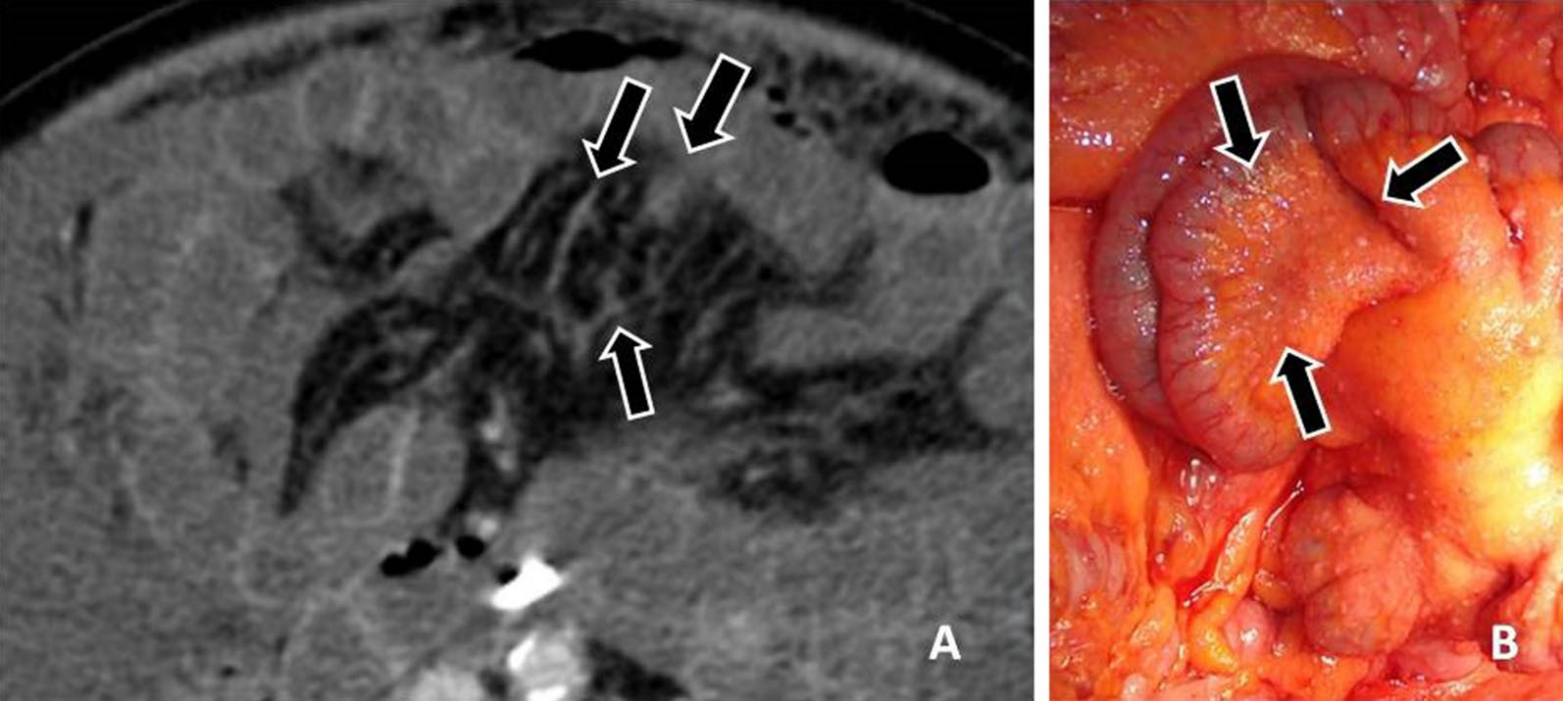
a CT enteroclysis image shows thickening of the mesenteric surface (arrows) and distortion and thickening of the fatty mesenteric folds. b At surgery multiple small implants on the mesentery are revealed (arrows)
